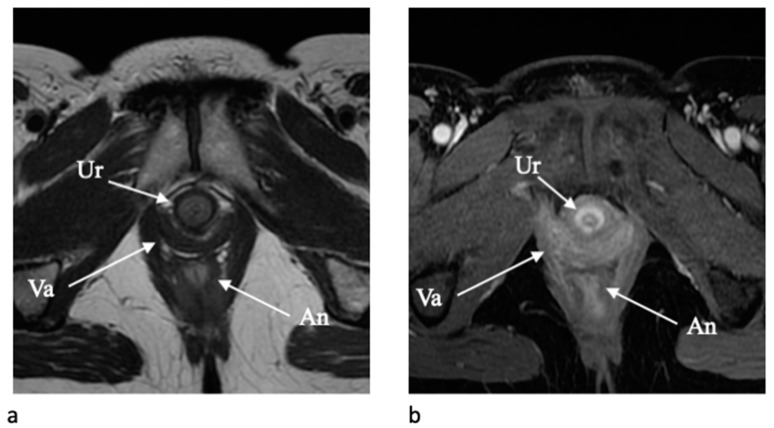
MR images of the urethra. Axial T2-W MR image (a) shows a typical target-like appearance of urethra (Ur), with an outer low-signal-intensity ring of external skeletal muscle of the urethral sphincter and a high signal intensity inner layer of smooth muscle and submucosa. Axial contrast-enhanced T1-W MR image (b) shows the corresponding aspect of the urethra (Ur) after contrast administration with evidence of an outer ring of tissue with less enhancement, representing the external skeletal muscle of the urethral sphincter, surrounding a circular band of higher enhancement of smooth muscle and submucosa. Posteriorly there are the vagina (Va) and the anus (An).
